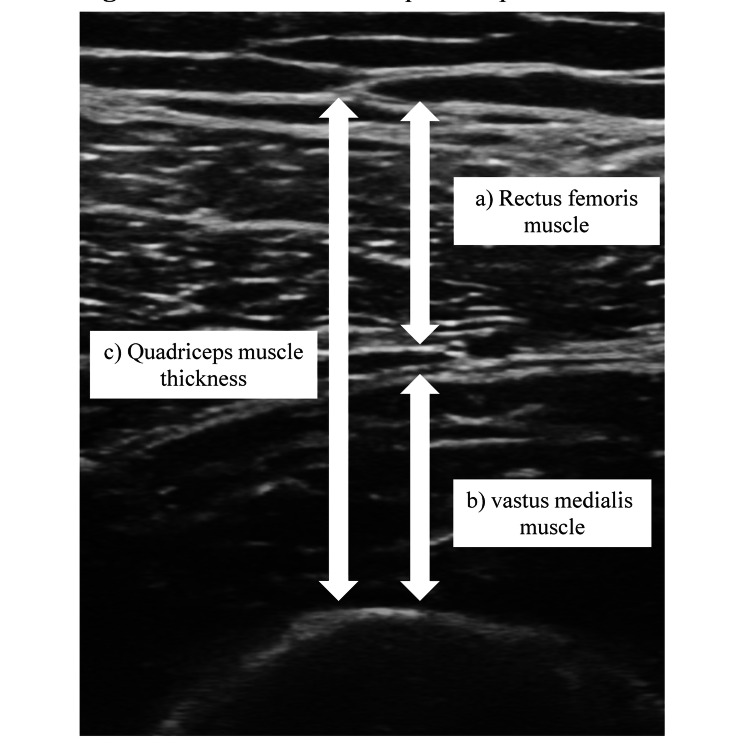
Measurement of quadriceps muscle thickness using an ultrasound imaging system ﻿﻿﻿(a) Rectus femoris muscle thickness. (b) Vastus medialis muscle thickness. (﻿﻿﻿c) Quadriceps muscle thickness (a + b).
